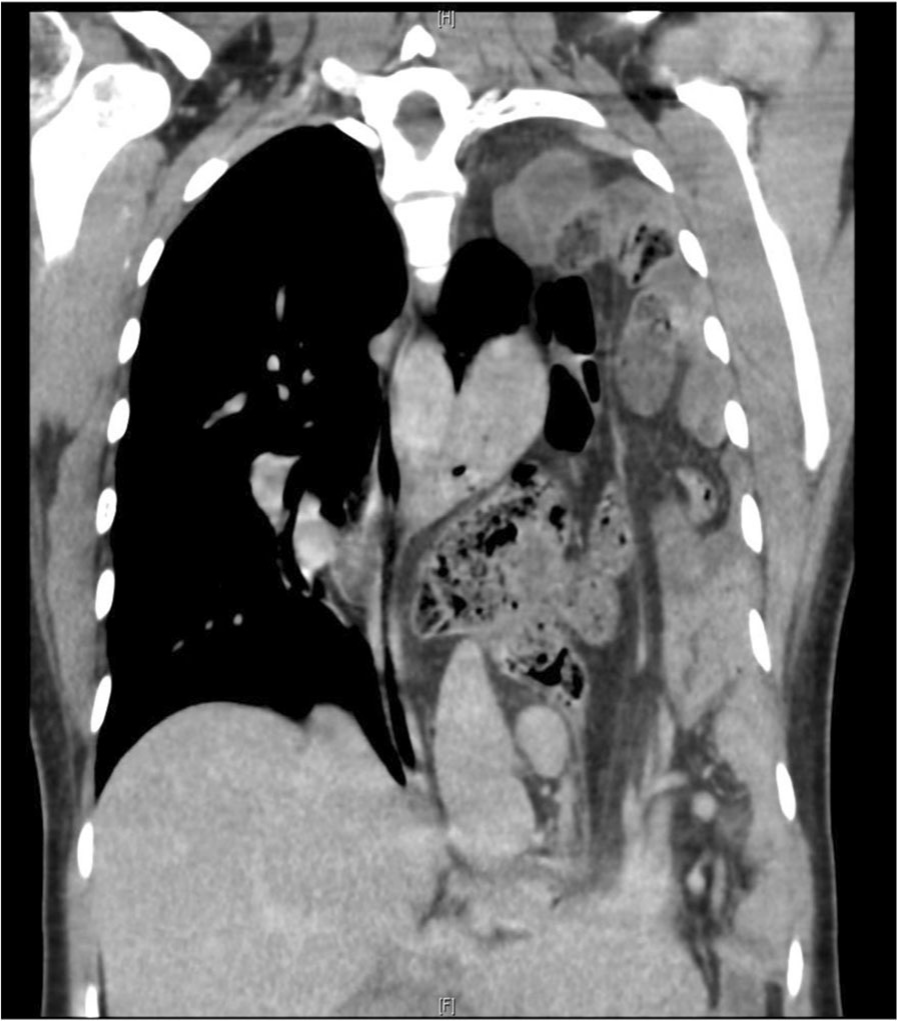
CT chest confirming a large diaphragmatic defect with herniation of stomach, small and large bowel and spleen into the chest cavity and almost complete collapse of the left lung
